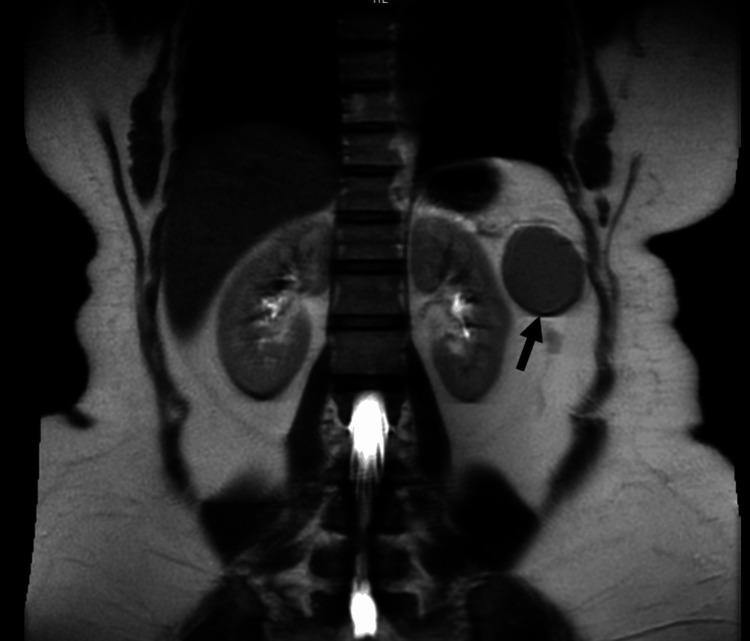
Abdominal MRI (coronal single-shot fast spin-echo (SSFSE) image) demonstrating a well-defined, homogeneous lesion (arrow) in the splenic fossa adjacent to the upper pole of the left kidney, showing signal intensity comparable to splenic tissue, suggestive of splenosis
